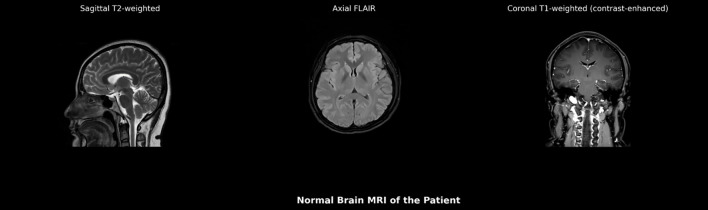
Normal brain MRI of the patient. (Left) Sagittal T2-weighted image; (Middle) Axial FLAIR image; (Right) Coronal T1-weighted contrast-enhanced image. No structural abnormalities were identified on any sequence.
